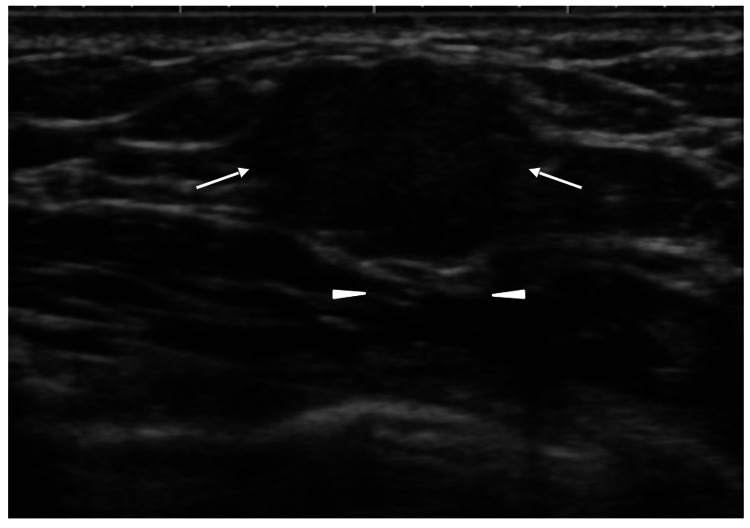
Ultrasound findings. Ultrasound showed an oval mass (arrows) with distinct borders, low internal echoes, punctate echogenic foci throughout the mass, and a faint posterior echo enhancement (arrowheads).
